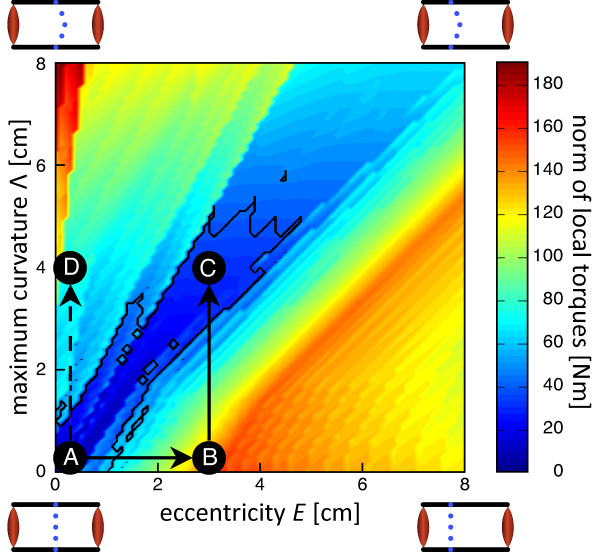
Norm of the local joint torques ||*CT|| *necessary for an equilibrium at each of the five lumbar spinal segments, as a function of the geometrical arrangement of the spine, according Equation 1. The contours enclose the spinal arrangements where the local joint torques do not exceeding ±30 Nm at any spinal segment. Position A - D: see Figure 3.
